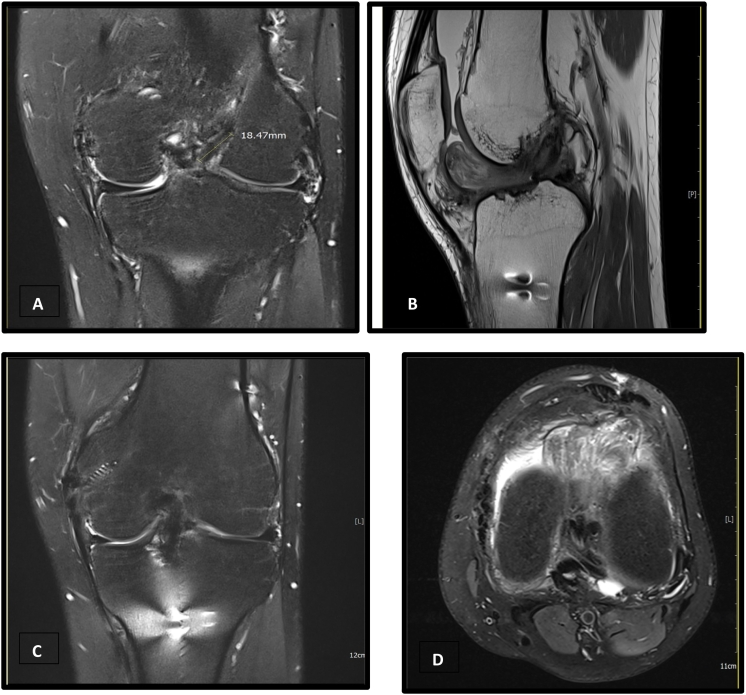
A–D, MRI examination showing cyclops lesion. A, MRI coronal T2 weighted image showing cyclops lesion size of 18.47 mm; B, medial extension of cyclops lesion; C, MRI showed intact ACL with MCL repair seen; D, axial view showing position of cyclops lesion at the intercondylar notch.
